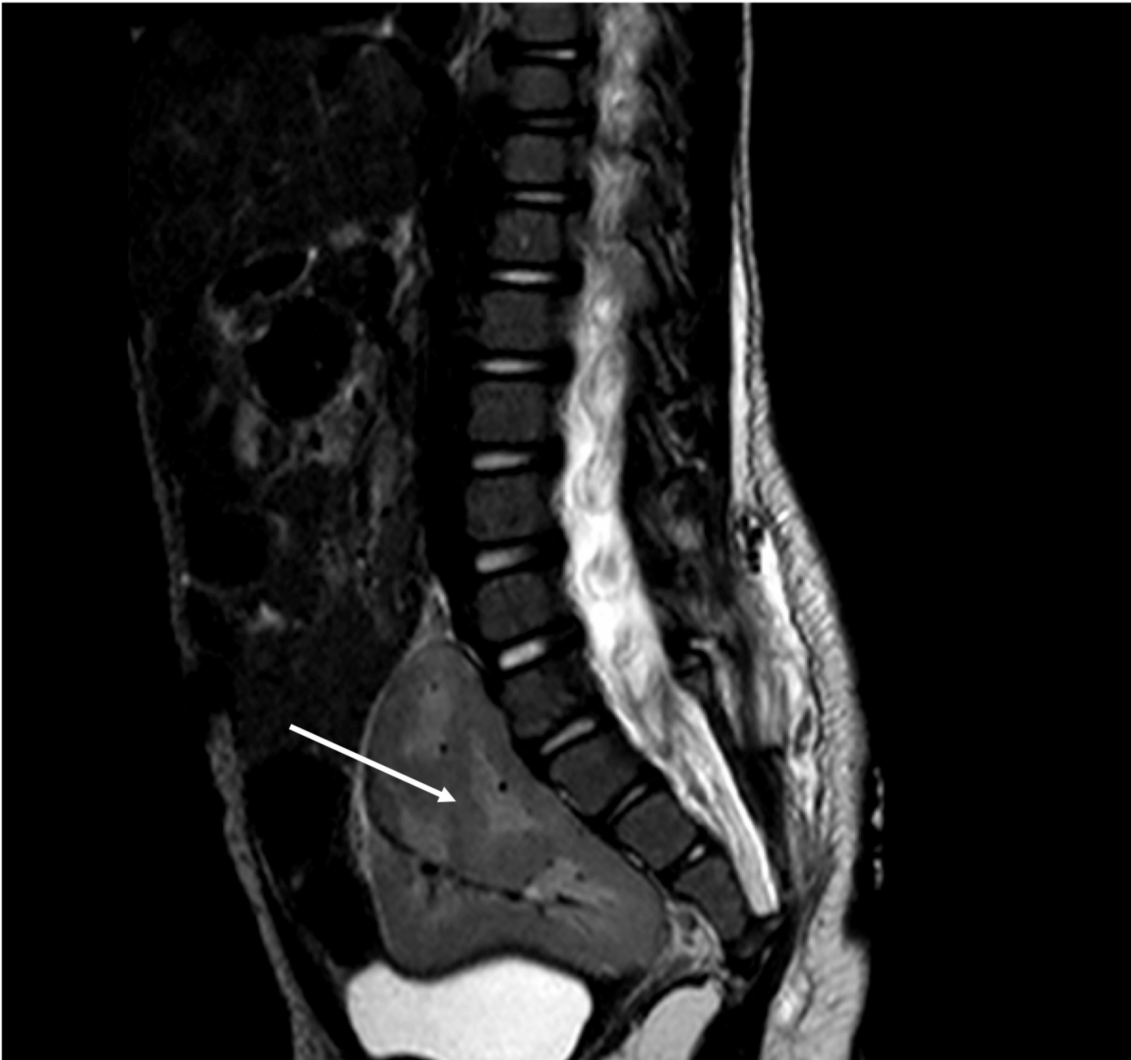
Sagittal T2-weighted magnetic resonance imaging (MRI) of the spine noting a pelvic kidney (arrow) This variant was found incidentally during the evaluation of a cutaneous flat capillary hemangioma, over the midline lumbar spine, to rule out occult spinal dysraphism. The patient had no history of pelvic pain or renal dysfunction.
